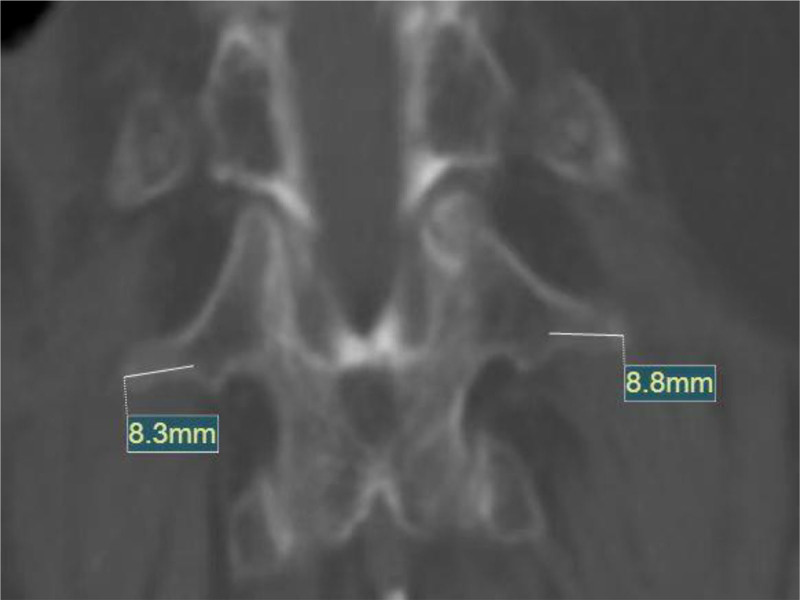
Preoperative CT reconstruction revealed lengths of 8.3 mm for the right L1 transverse process and 8.8 mm for the left.
